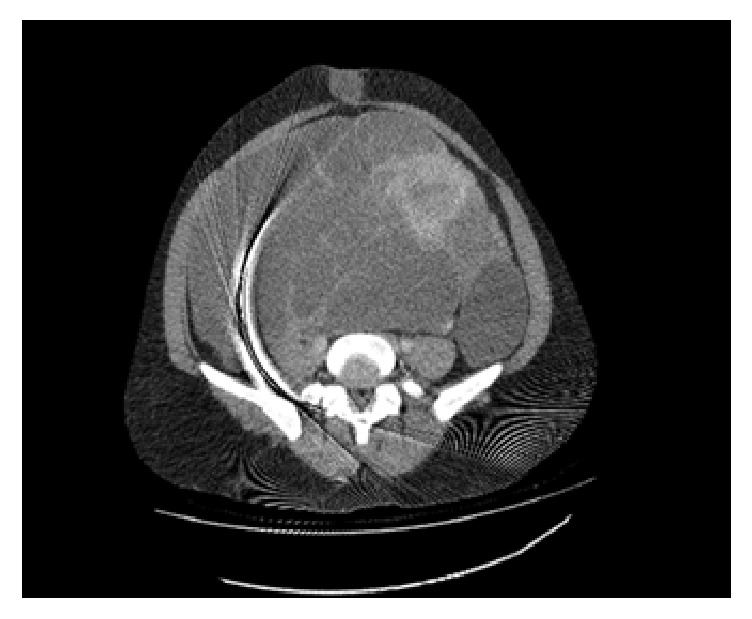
Intravenous contrast CT scan showing a large septated solid-cystic mass with report of serous cyst adenoma.
